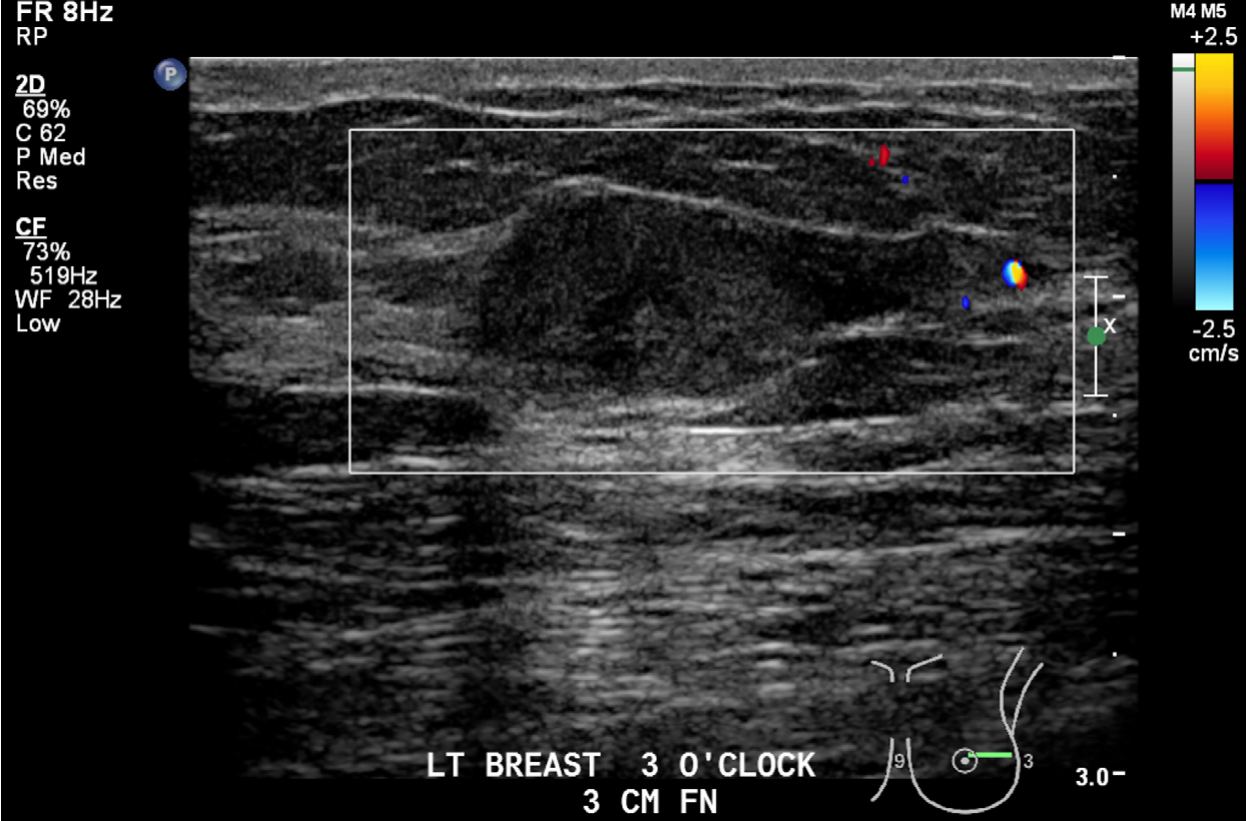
Lesion one with Doppler interrogation demonstrating mild vascularity. Image courtesy of BreastScreen ACT.
